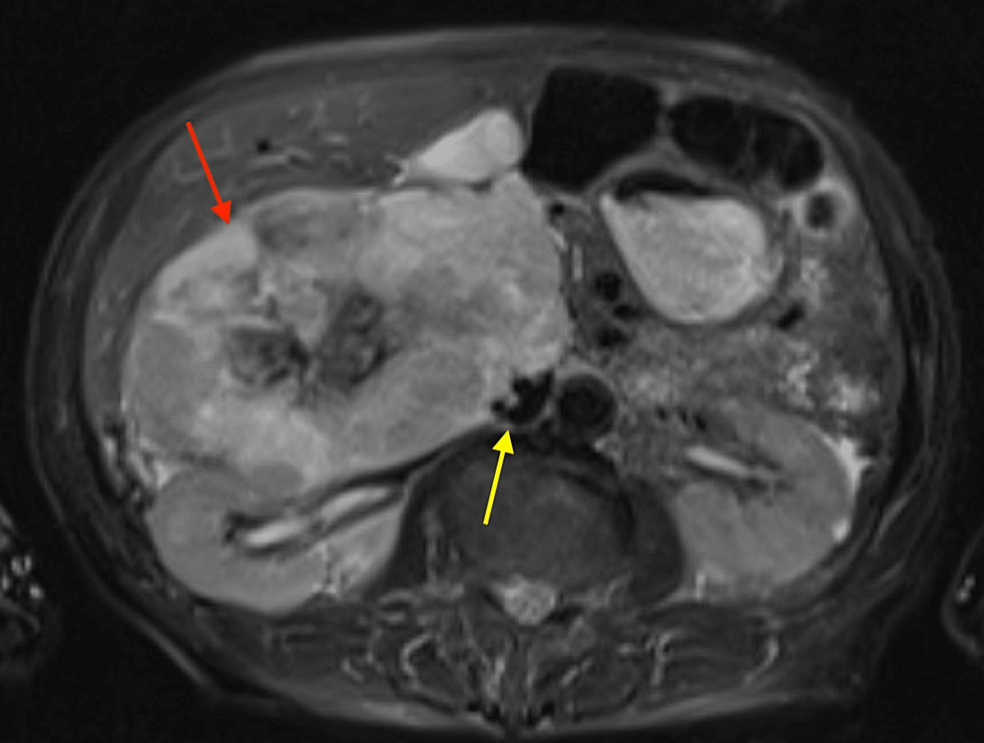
MRI adrenals with and without contrast axial view revealing a large, heterogeneously enhancing mass (red arrow) seen abutting and compressing the inferior vena cava (yellow arrow).
